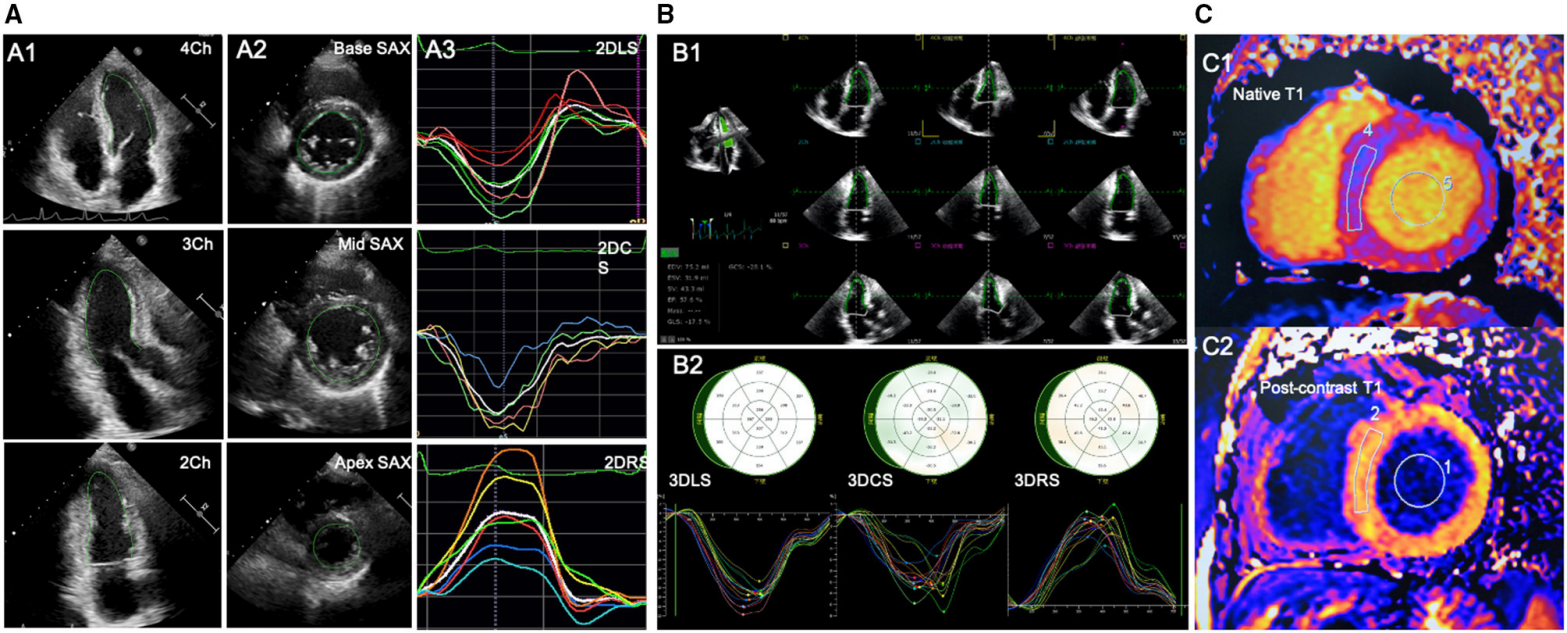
Offline analysis of STE for left ventricular (LV) strain and CMR for LV-ECV. (A) 2D-STE offline analysis for LV. A1-A2, LV endocardial tracing in the three LV apical long-axis and three LV short-axis views; A3, LV strain curves; (B) 3D-STE offline analysis for LV. B1, LV endocardial tracing at end-diastole and end-systole; B2, 3D-GLS, GCS, and GRS strain curves were generated. (C) Offline analysis for CMR-LV-ECV. C1-C2, measurements of native and postcontrast T1 time from LV myocardium and LV blood pool. 2D-STE, two-dimensional speckle-tracking echocardiography; 3D-STE, three-dimensional STE; CMR, cardiovascular magnetic resonance; ECV, extracellular volume fraction; GLS, global longitudinal strain; GCS, global circumferential strain; GRS, global radial strain.
